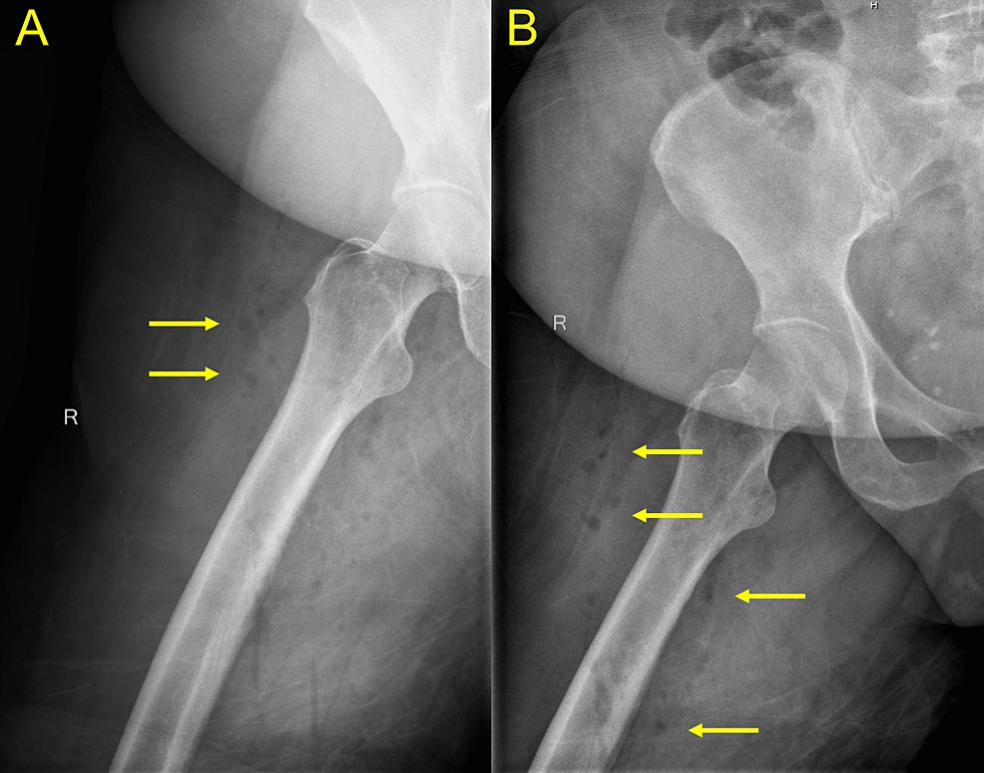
Standard roentgenography of the right lower extremity Plain films of the right lower extremity demonstrate gas in the deep tissues of the right thigh (yellow arrows). (A) Lateral view. (B) Anteroposterior view.
